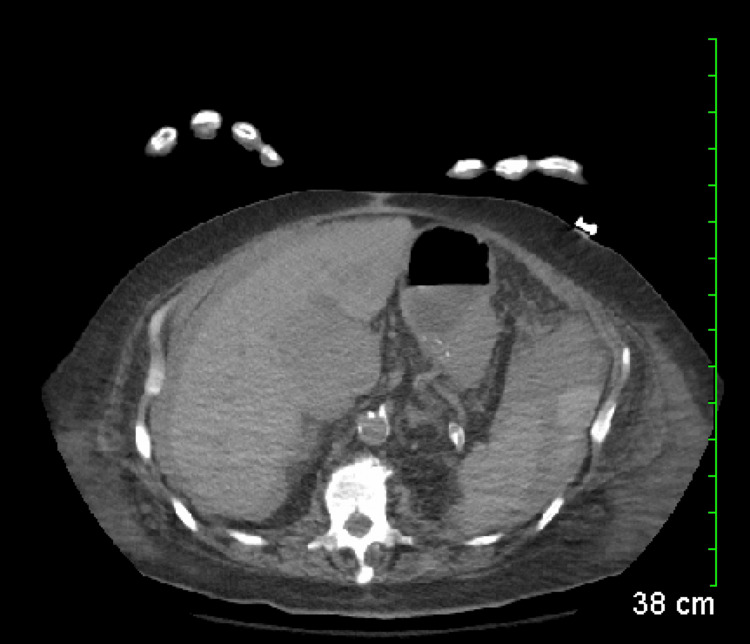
CT of the abdomen without contrast Multiple indeterminate low-density hepatic lesions, the largest measuring 7.5 cm. There is likewise moderate volume ascites seen
